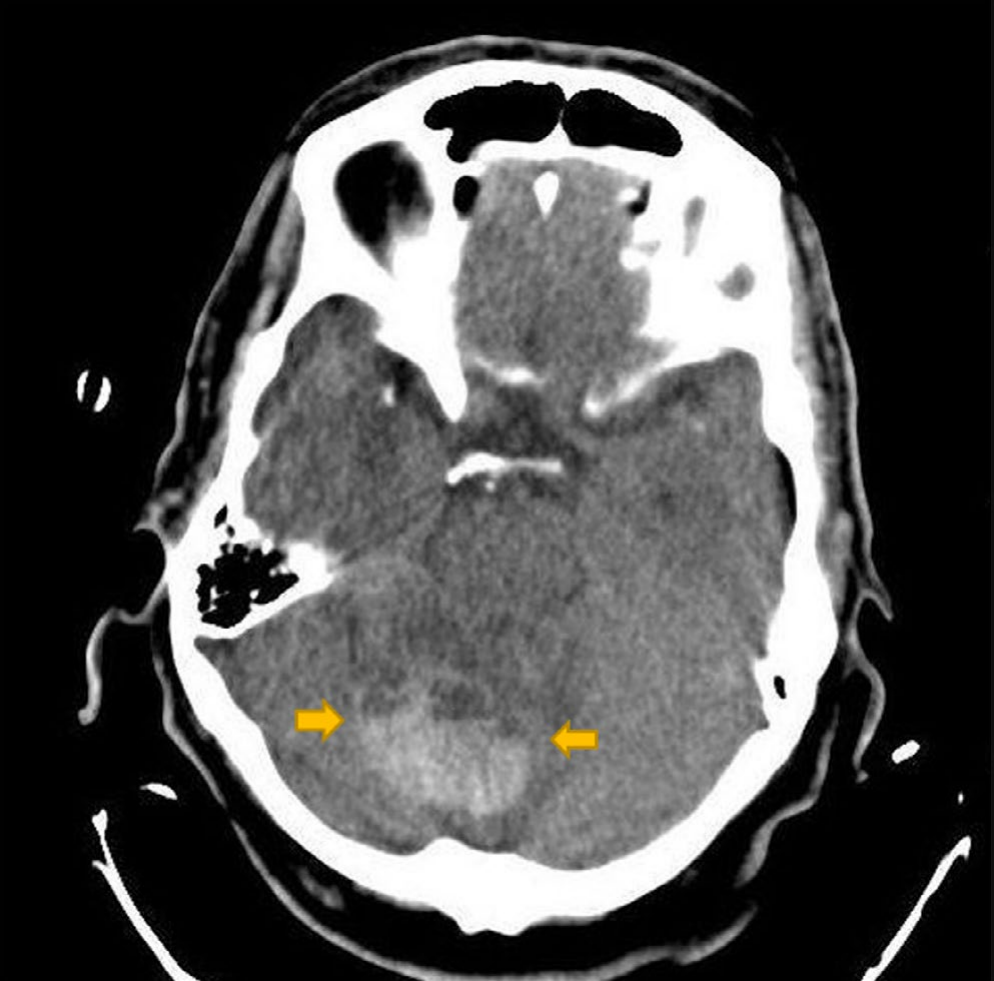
Right cerebellar hemorrhagic mass with peripheral edema in CT scan without contrast
